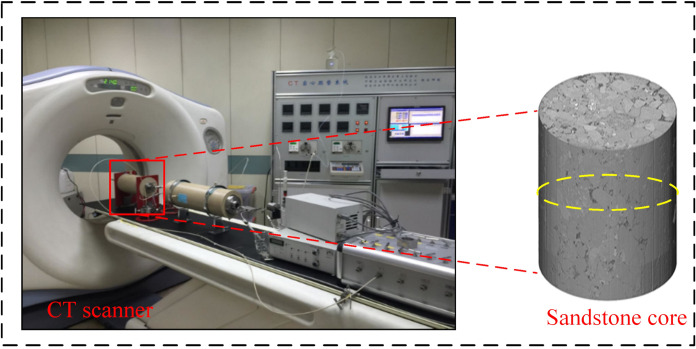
The CT scan of the core.
